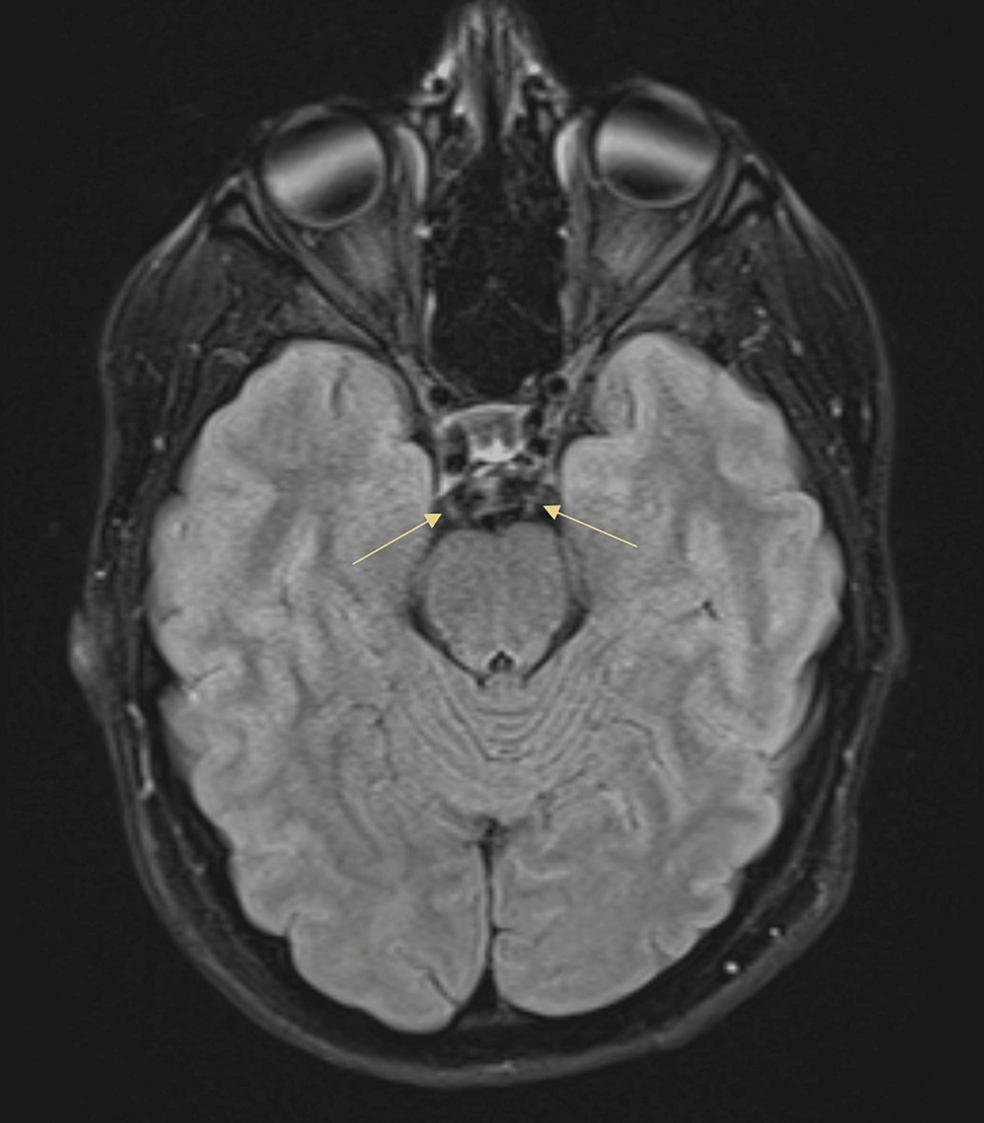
FLAIR axial image of brain MRI showing no enhancement of oculomotor nerves (yellow arrows).
